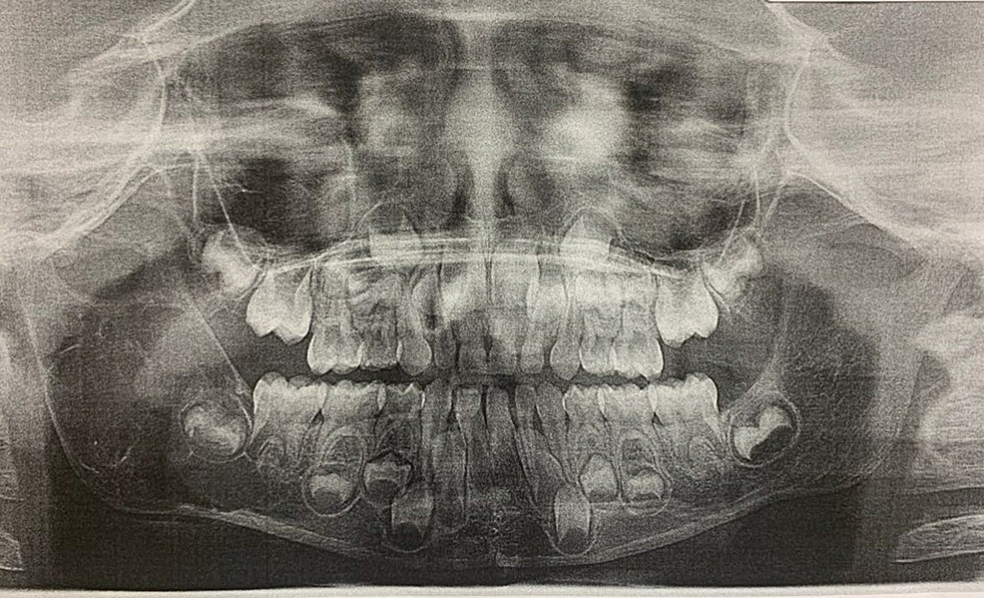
Panoramic radiograph with mandibular lesion
